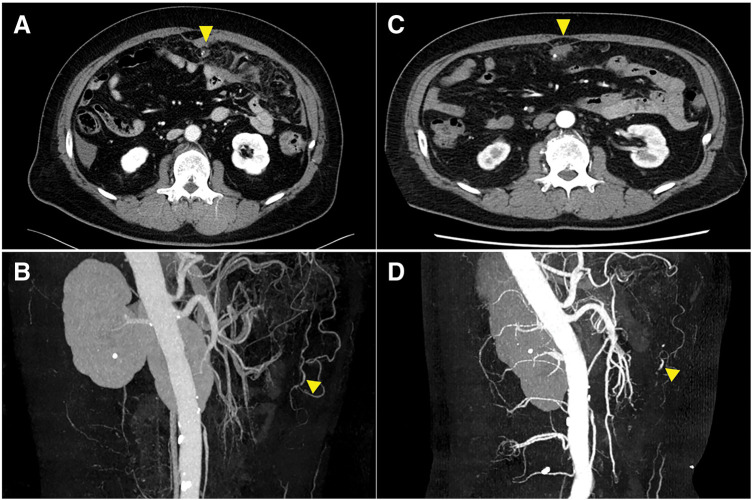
Images of contrast-enhanced CT. (A, B) Contrast-enhanced CT revealed a hematoma and an aneurysm in the omentum (triangle). (C, D) The aneurysm has grown slightly in size in 7 days (triangle).
